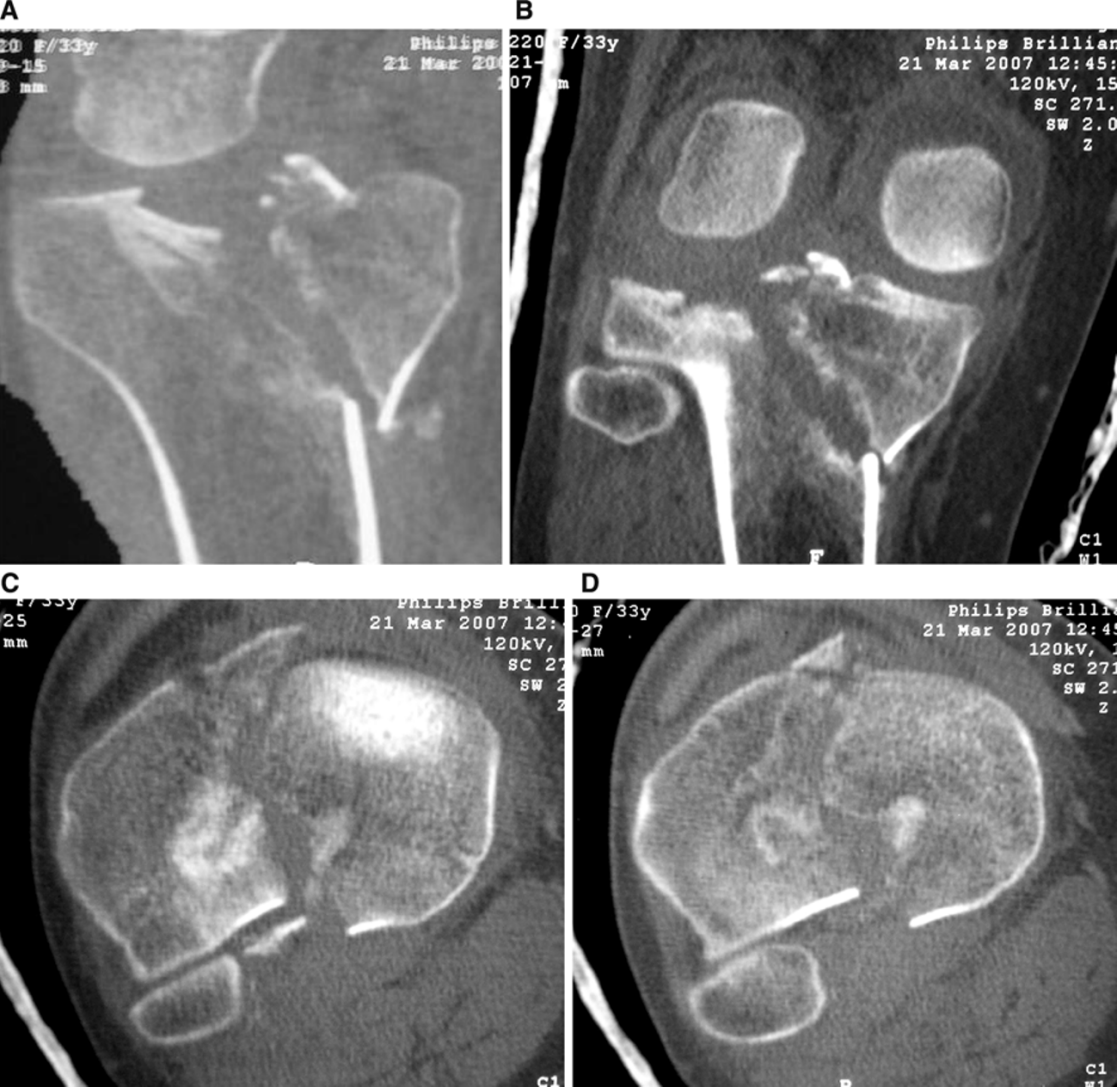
a Schatzker type IV fracture, CT scan. b Schatzker type IV fracture, CT scan. c Schatzker type IV fracture, CT scan. d Schatzker type IV fracture, CT scan
